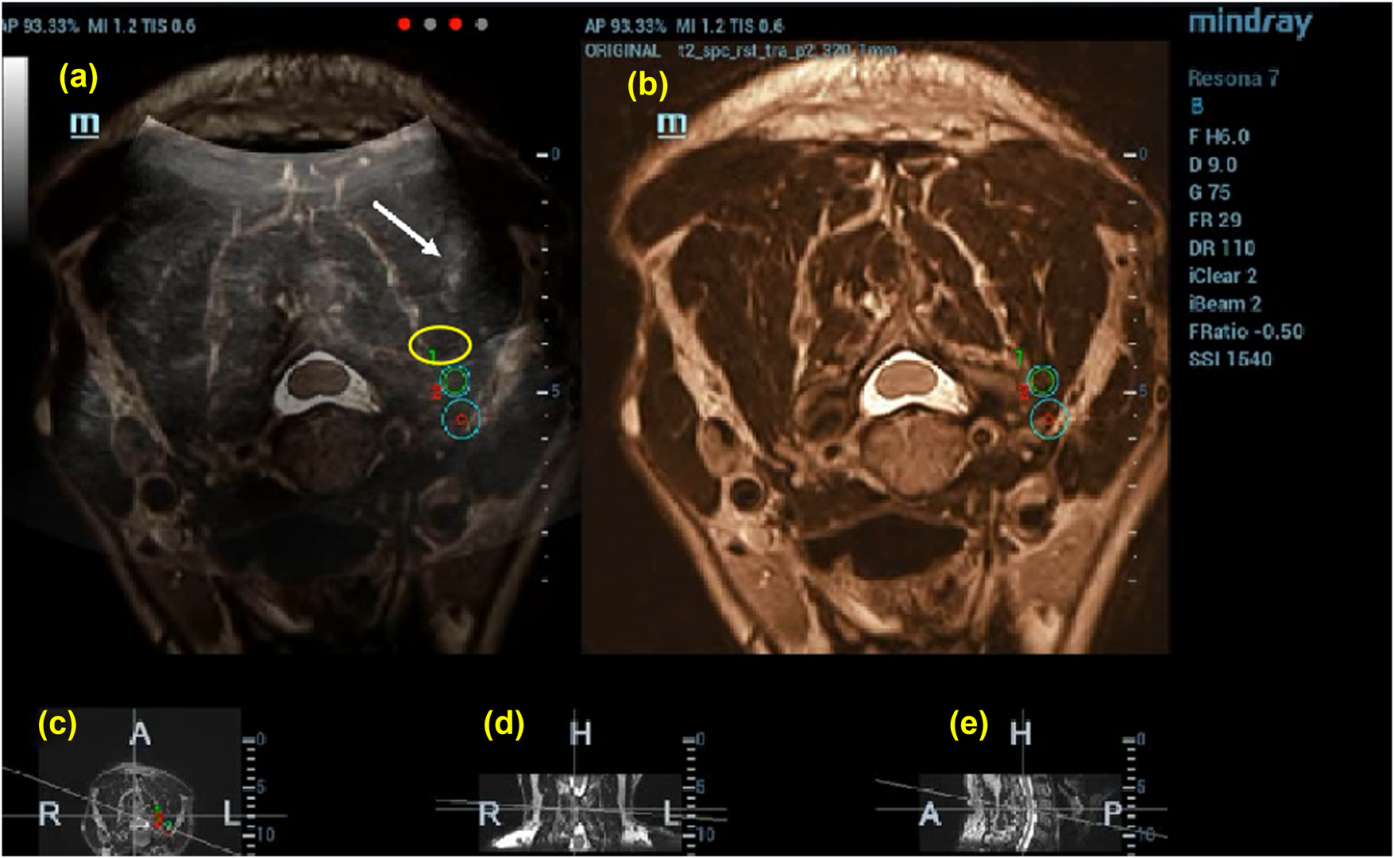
Puncture to preset target 1 guided by US (50%)-MRI (50%) image. The drug solution is injected after the puncture is guided by US (50%)-nuclear magnetic resonance (50%) image; the white arrow indicates the location of the puncture needle and the yellow circle indicates the solutions diffusion range. (a) US-MRI fusion images, with green circles indicating the target puncture points, (b)MRI images, (c) cross section, (d) coronal section, and (e) sagittal section.
